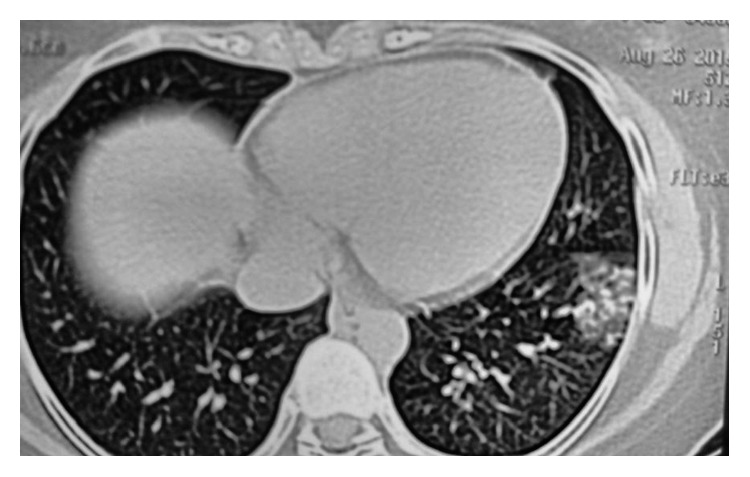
HRCT showing recurrence of bilateral patchy lesions in different zones.
